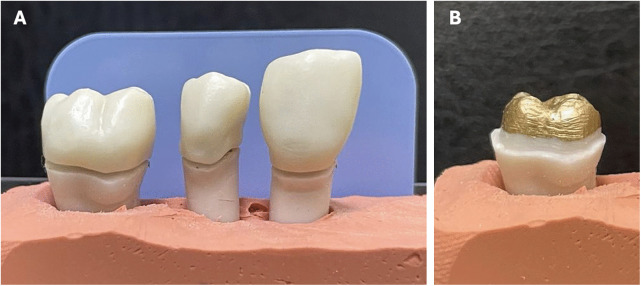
Preparation of model teeth. (A) Embedding of model teeth in a putty index for radiography. (B) Application of a layer of 14-micron dye spacer to the prepared teeth, maintaining a 1 mm distance from the margin.
